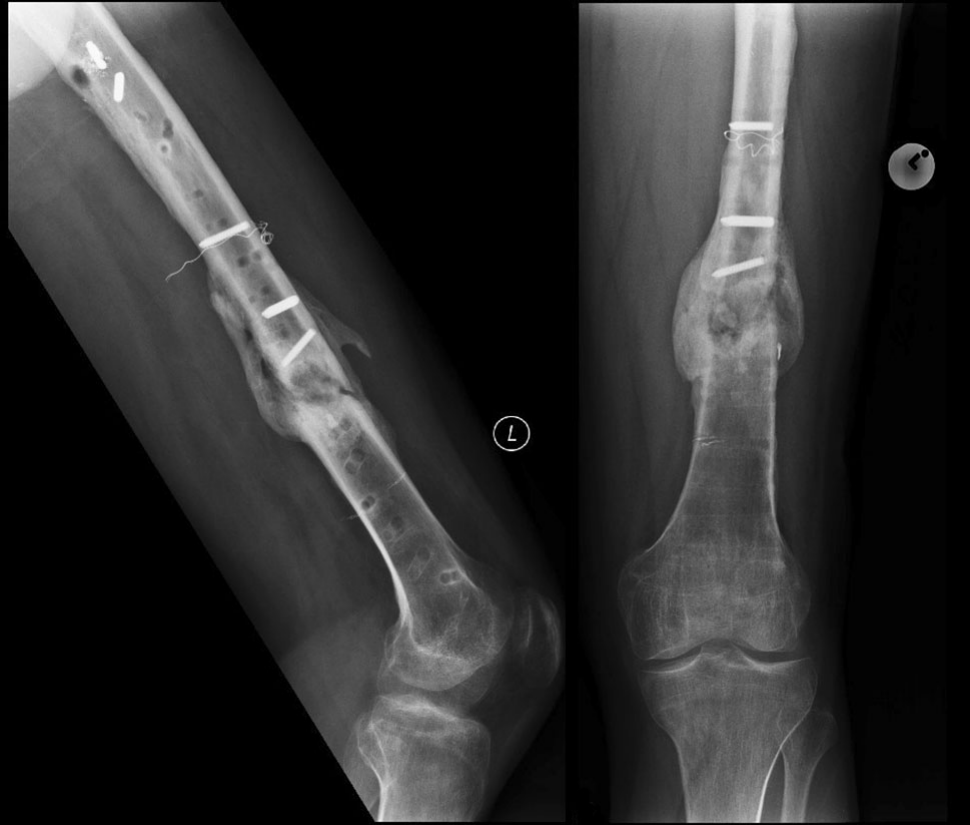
Anteroposterior and lateral radiographs of united femur after hexapod removal
